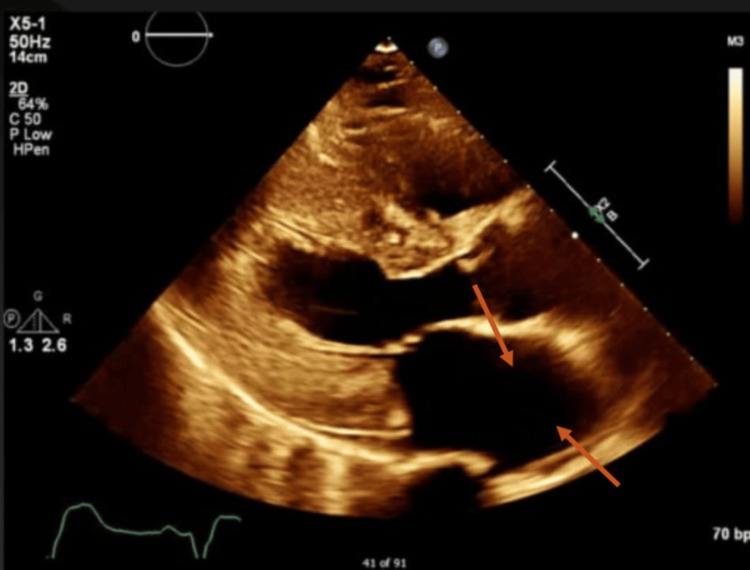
Transthoracic echocardiogram, parasternal long-axis view, showing left atrial enlargement (arrows) and mild diffuse hypokinesis
